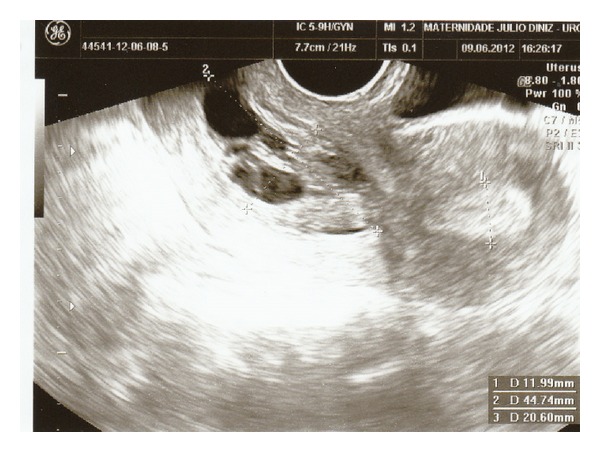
Endovaginal ultrasound: intracervical multicystic mass, with 45 mm in its maximum diameter.
